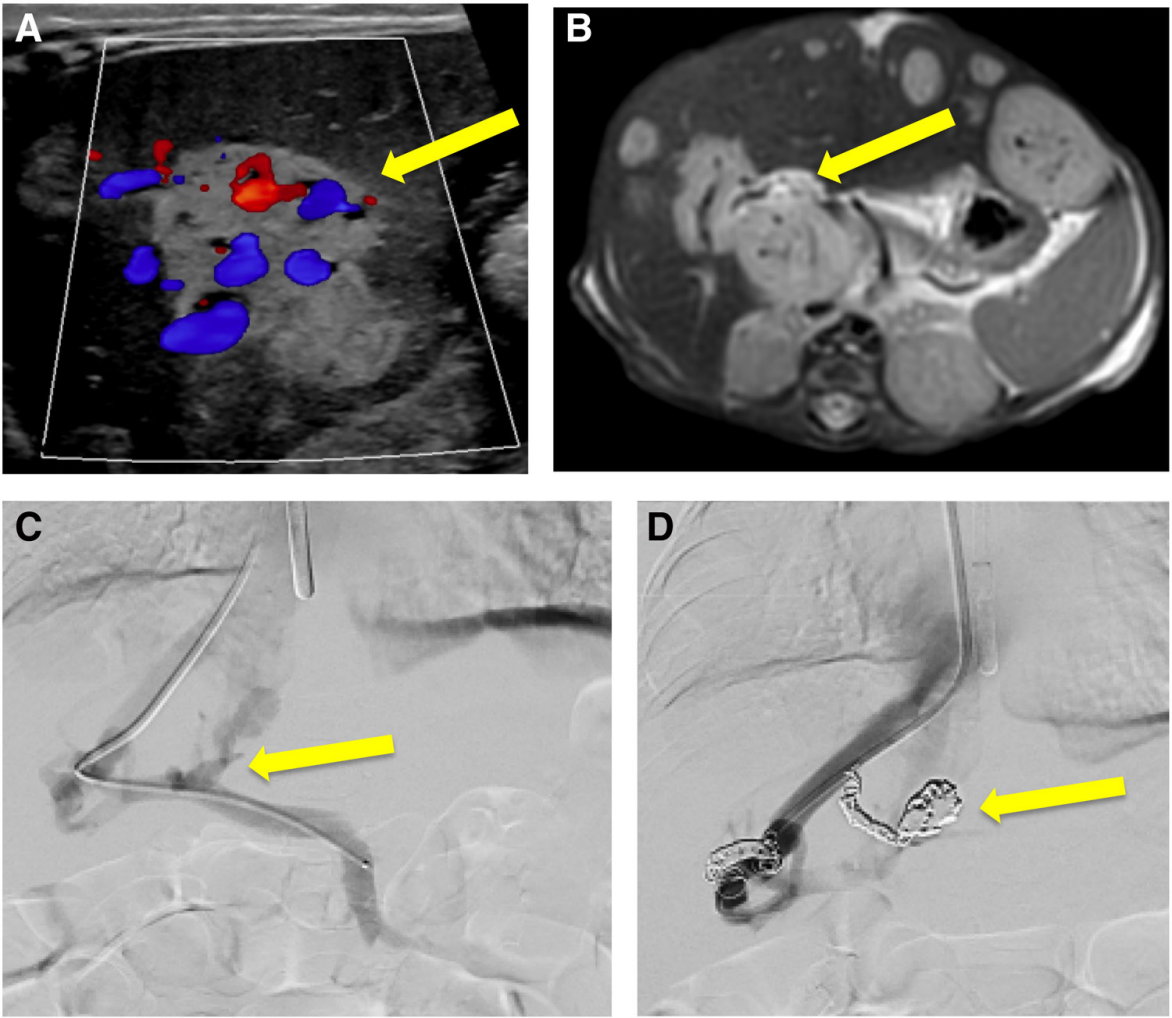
Imaging and embolization of multiple intrahepatic shunts within hepatic hemangiomas. A and B) Ultrasound with Doppler and MR imaging showed multiple hepatic hemangiomas containing portosystemic shunts (arrow). C and D) Coil embolization of four of the dominant portosystemic shunts (arrow) within hemangiomas was performed. MR = magnetic resonance.
